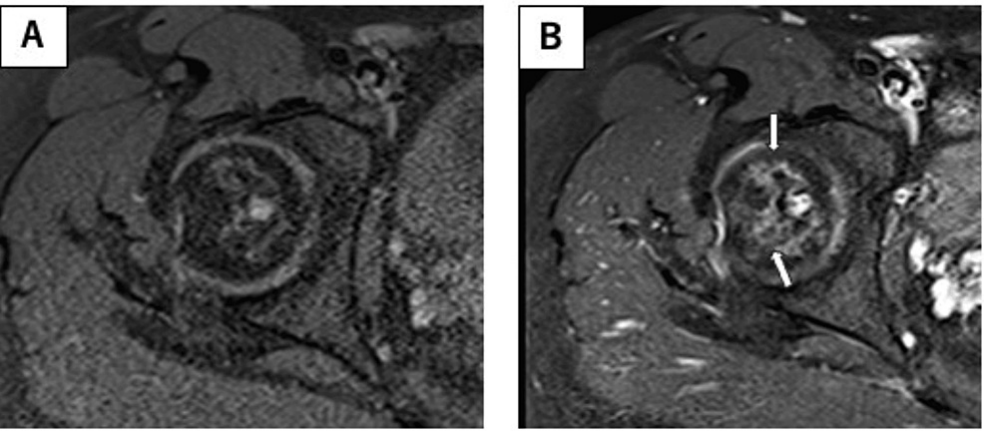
Gadolinium-enhanced magnetic resonance image taken 2.3 years postoperatively, revealing a partial contrast effect at the epiphysis (arrow). (A) Plain T1WI axial view; (B) gadolinium-enhanced T1WI axial view.
